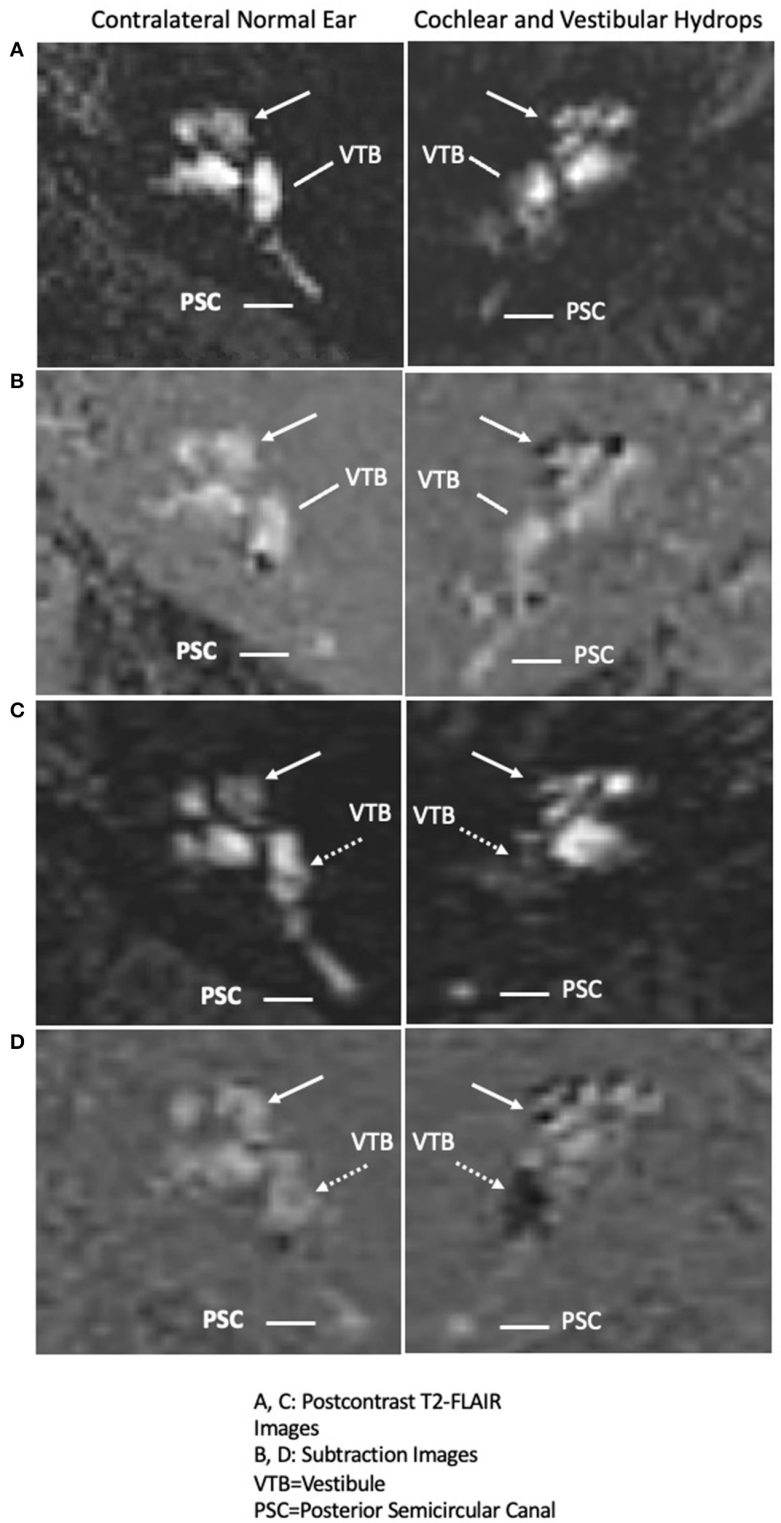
Interval progression of cochlear endolymphatic hydrops in 2015 to cochlear and vestibular endolymphatic hydrops in 2019 on 3T MRI: *Right* column: Endolymphatic hydrops and *Left* column: contralateral normal ear. (A) (Right panel: Cochlear hydrops in 2015 MRI), delayed postcontrast T2-FLAIR images through the cochlea demonstrate a prominent scala media signal void consistent with a dilated cochlear duct (arrow) in the right ear (Left panel: normal contralateral side), a normal appearing cochlea in the left ear. Posterior semicircular canal (PSC) and vestibule (VTB) are labeled for reference. (B) (Right panel: Cochlear hydrops in 2015 MRI), corresponding subtracted images more clearly isolate the cochlear duct delineated against a nullified background (Left panel: normal contralateral side), a normal appearing cochlea in the left ear. (C) (Right panel: Cochlear and Vestibular hydrops in 2019 MRI), delayed postcontrast T2-FLAIR images demonstrate a prominent cochlear duct and vestibule signal void consistent with cochlear (arrow) and vestibular (dashed arrow) hydrops in the right ear (Left panel: normal contralateral side), normal appearance of the cochlea and vestibule. (D) (Right panel: Cochlear and Vestibular hydrops in 2019 MRI), corresponding subtracted images more clearly isolate the cochlear duct (arrow) and vestibule (dashed arrow) against a nullified background in the right ear (Left panel: normal contralateral side), normal subtracted images of the cochlea and vestibule in the left ear.
